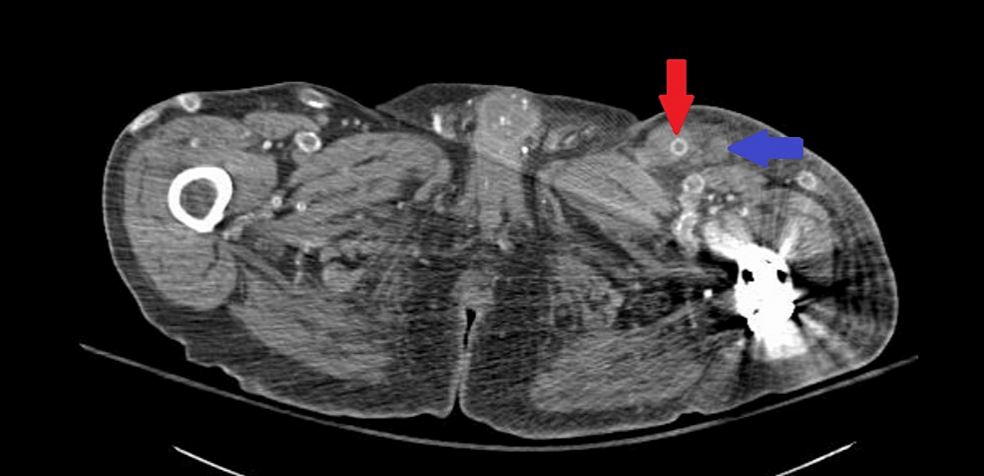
Axial computed tomography angiogram through the superior thigh demonstrating an intermediate-density fluid collection tracking along the venous limb of the arteriovenous graft with an adjacent reactive lymph node Red arrow: arteriovenous graft, blue arrow: lymph node
